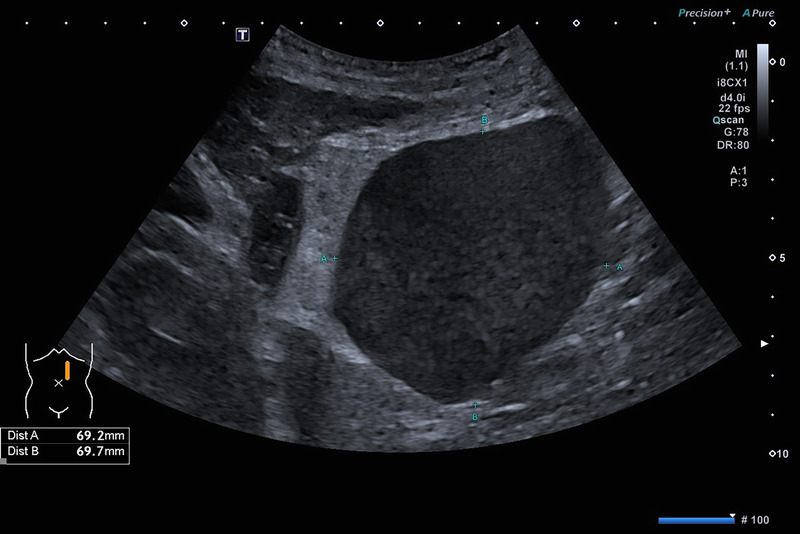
Abdominal ultrasound of the cystic lesion.
